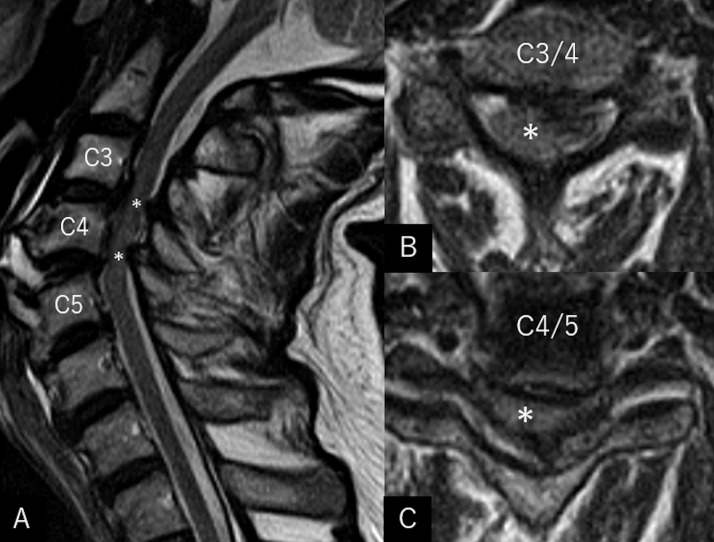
Sagittal (A) and axial (B, C) T2-weighted magnetic resonance images of the cervical spine showing severe cord compression at the C3/4 and C4/5 levels (asterisk).
